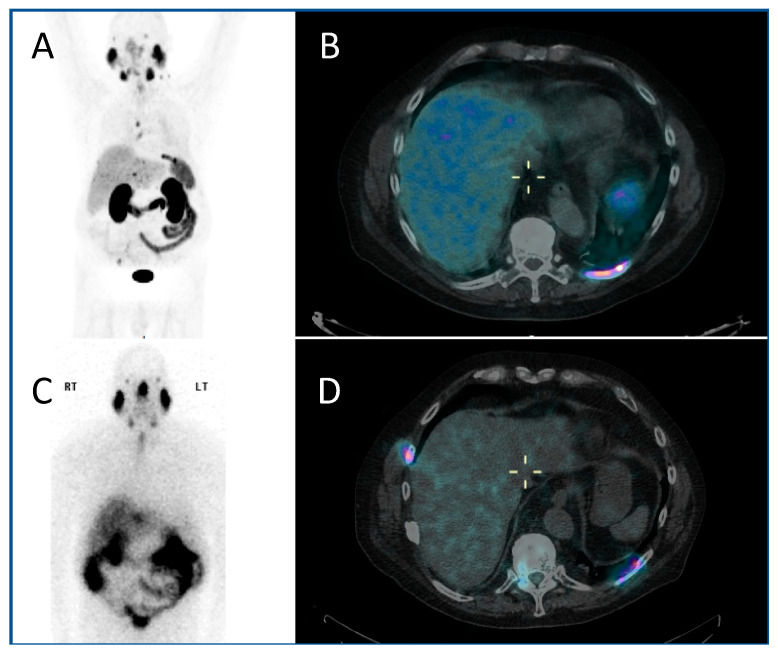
A PMSA-based theragnostic approach to metastatic prostate cancer. (A) Anterior view of maximum intensity projection, and (B) axial view of ^68^Ga-PMSA PET CT (positron emission tomography, computed tomography) demonstrating left posterior rib metastasis. Then, following ^177^Lu PMSA administration, (C) anterior view planar scintigraphy and (D) SPECT CT (single photon emission computed tomography, computed tomography) imaging. While these show similar appearances, post-therapy imaging has a lower resolution as the techniques are different.
